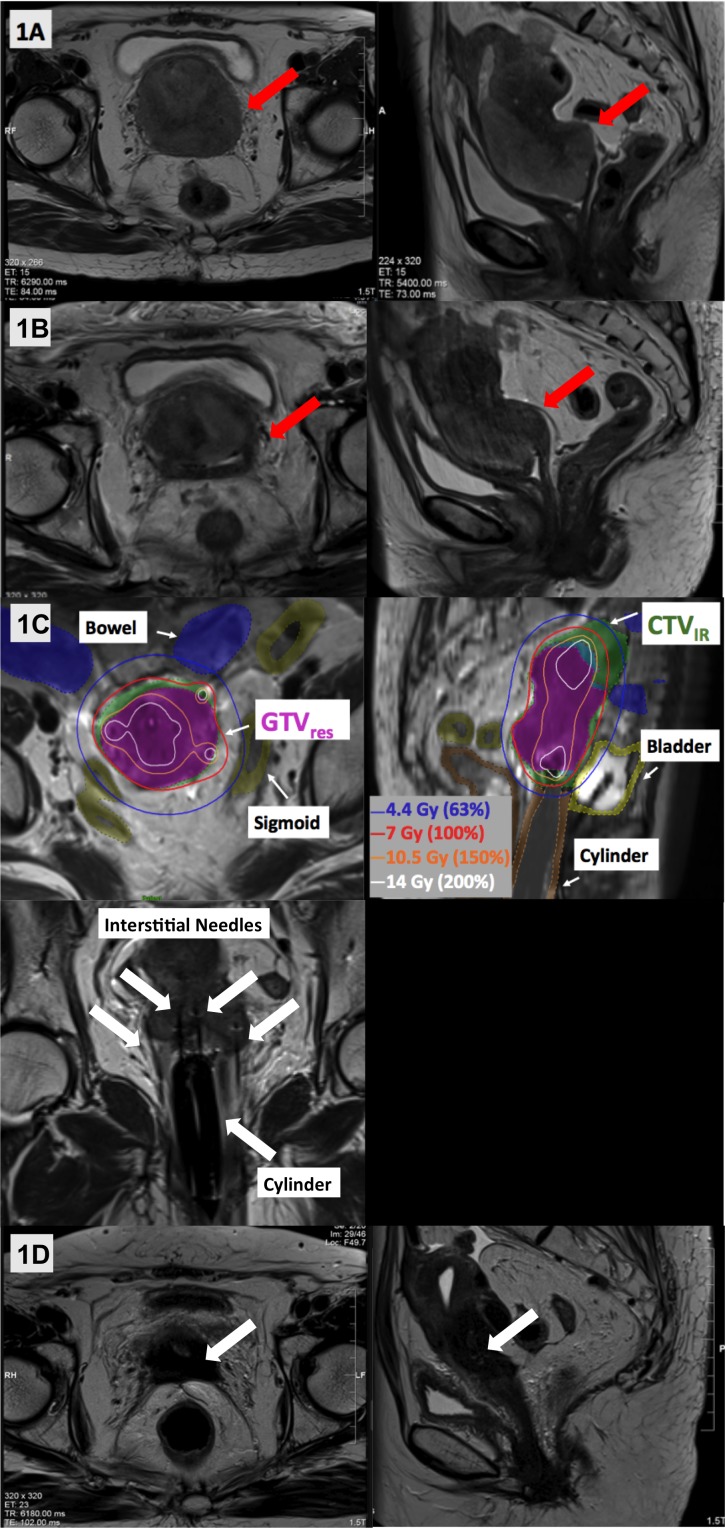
Representative T2-weighted axial and sagittal images acquired across Patient A’s treatment course. (A) Pre-treatment MRI showed a large uterine mass involving the cervix, vagina, and left parametria (red arrows). (B) MRI following external beam radiotherapy showed a good response with reduction in the size of the mass, but persistent parametrial involvement rendering it still unresectable (red arrows). (C) MR-guided brachytherapy was delivered using a template, intra-vaginal cylinder and 12 interstitial needles, with good dosimetric coverage of the residual GTV (GTVres: solid magenta), high-risk CTV (CTVHR: overlaps with GTV res) and intermediate risk CTV (CTVIR: solid green). (D) MRI at 14 months post-brachytherapy showed a complete response, with fibrosis and no residual tumor (white arrows). CTV_HR_ = High risk clinical target volume; CTV_IR_ = Intermediate risk clinical target volume; GTV_res _= Residual gross tumor volume at brachytherapy
